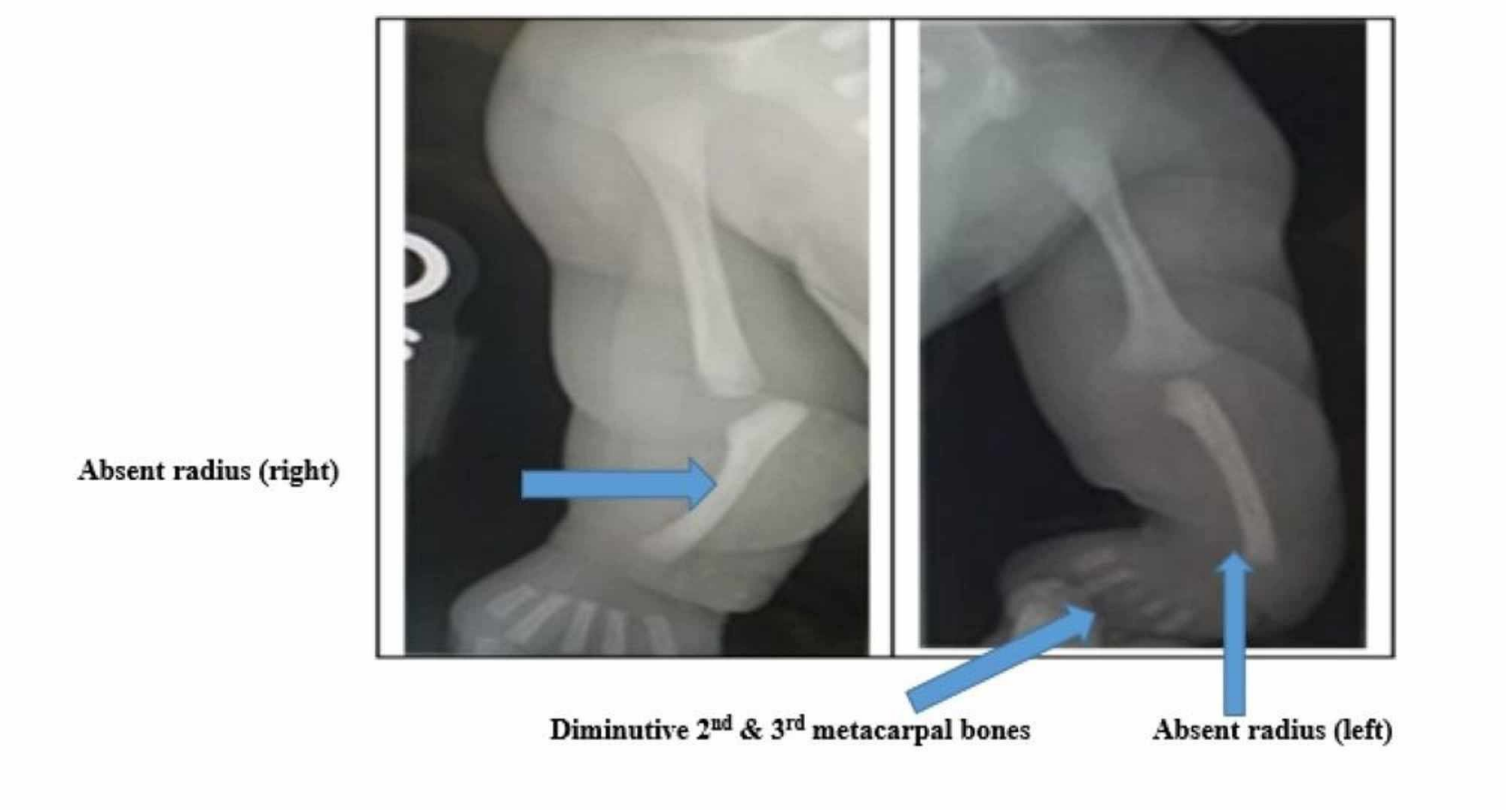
X-ray of upper extremities demonstrating bilateral absence of the radii
